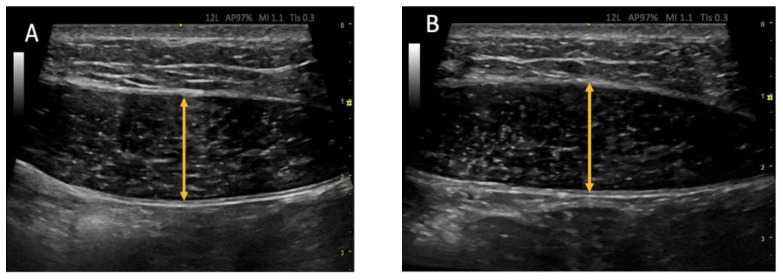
Ultrasonic images of the rectus abdominis (RA). (A) at rest and (B) during muscle activity. Measurements are taken between the internal borders of the widest area of the muscle belly. An increase in muscle thickness is appreciable during contraction (B).
